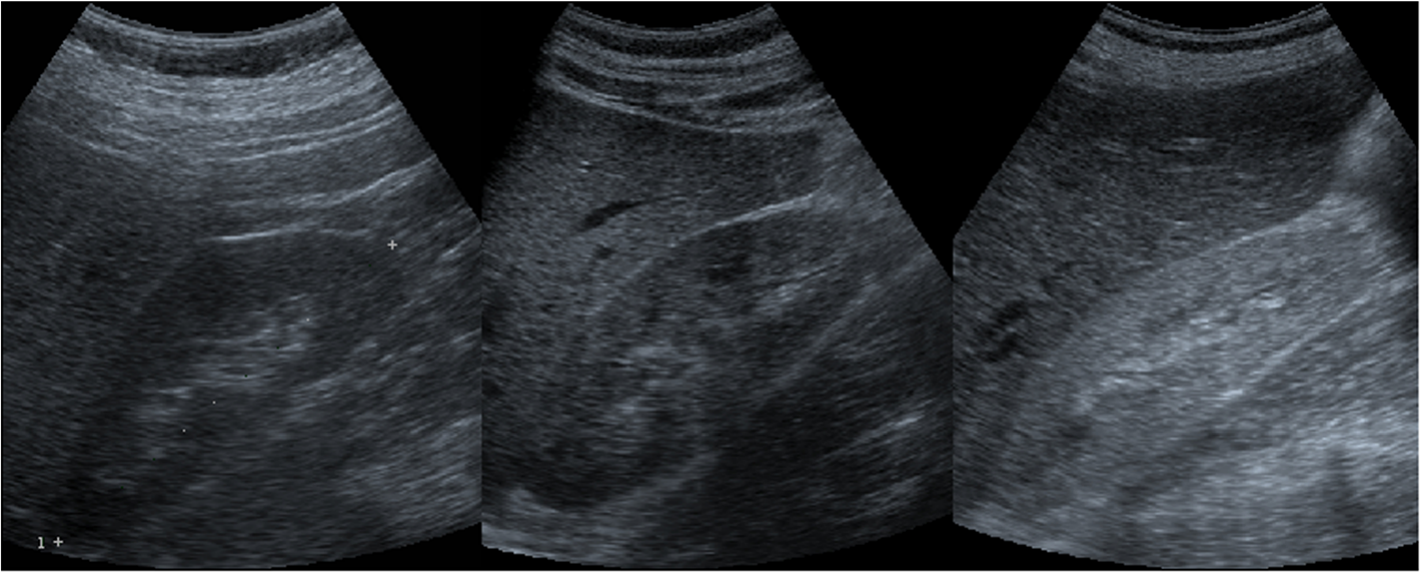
Sonograms were graded by the degree of cortical echogenicity relative to the liver or spleen parenchyma in the following manner: Left: less than (grade 0); Middle: equal to (grade 1); Right: greater than (grade 2)
